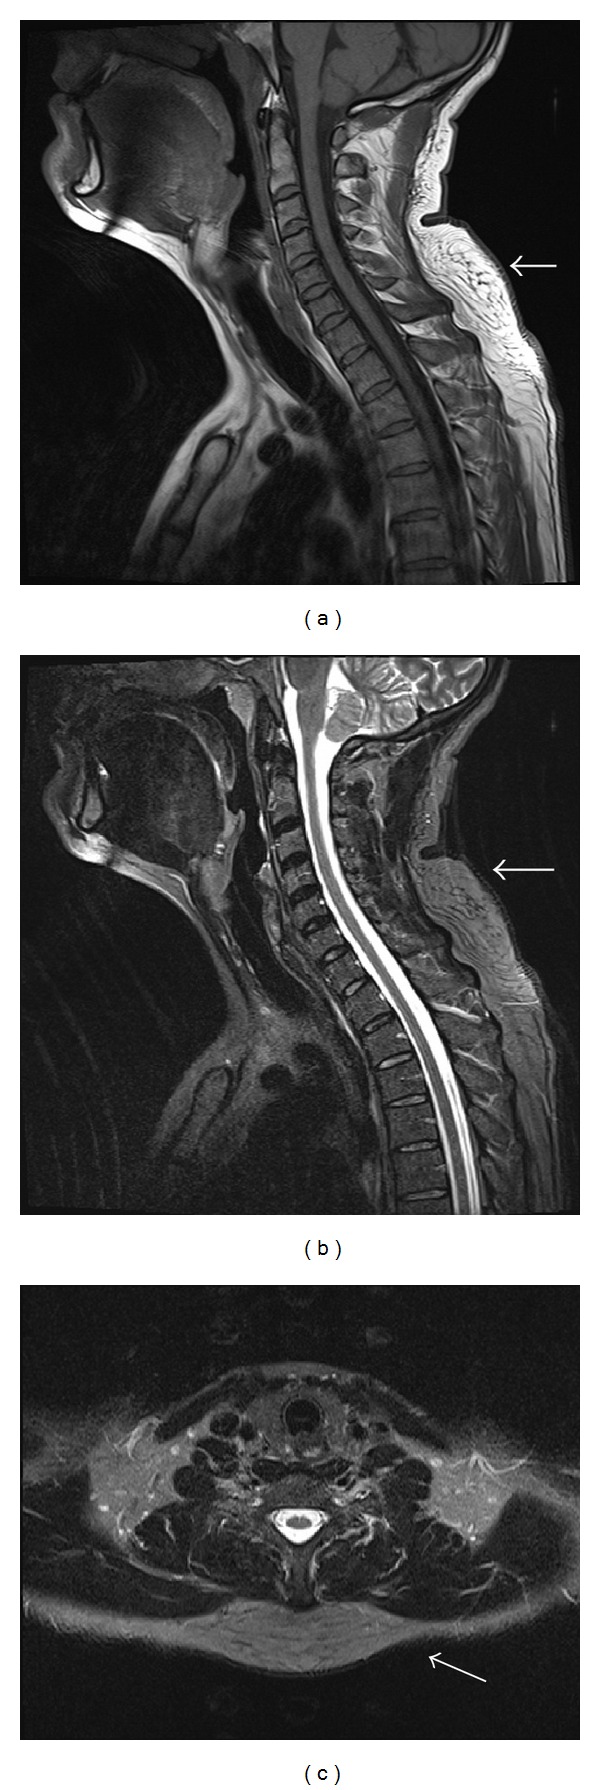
MRI of the neck demonstrates a subcutaneous mass (white arrows) in the interscapular region isointense to surrounding subcutaneous fat on T1-weighted sequence (Figure 3(a), repetition time/echo time = 451/11 msec) and on fat-suppressed sequence (Figures 3(b) and 3(c), repetition time/echo time = 7090/89 msec). There is absence of a low signal intensity fibrous capsule (Figure 3(c)) typical of a lipoma.
